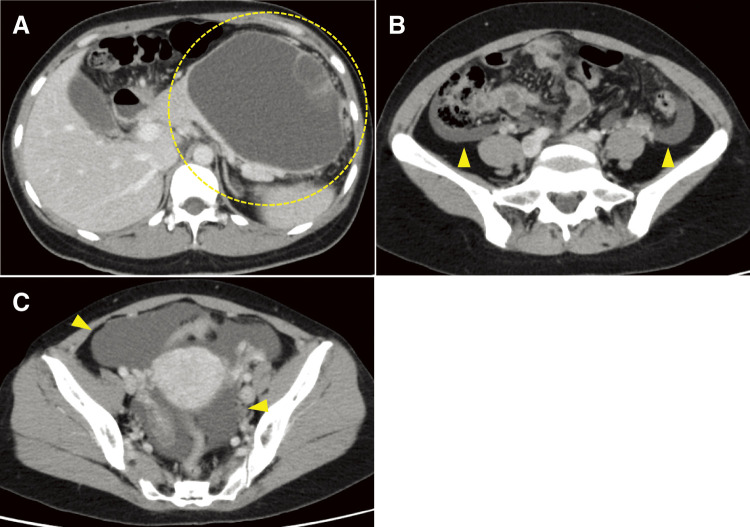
The CECT revealed findings upon the patient’s visit to our emergency department: (A) A shrinking cystic mass in the pancreatic tail (dotted line). (B, C) Ascites from the paracolonic groove to the pelvic region (arrowheads). CECT, contrast-enhanced computed tomography
